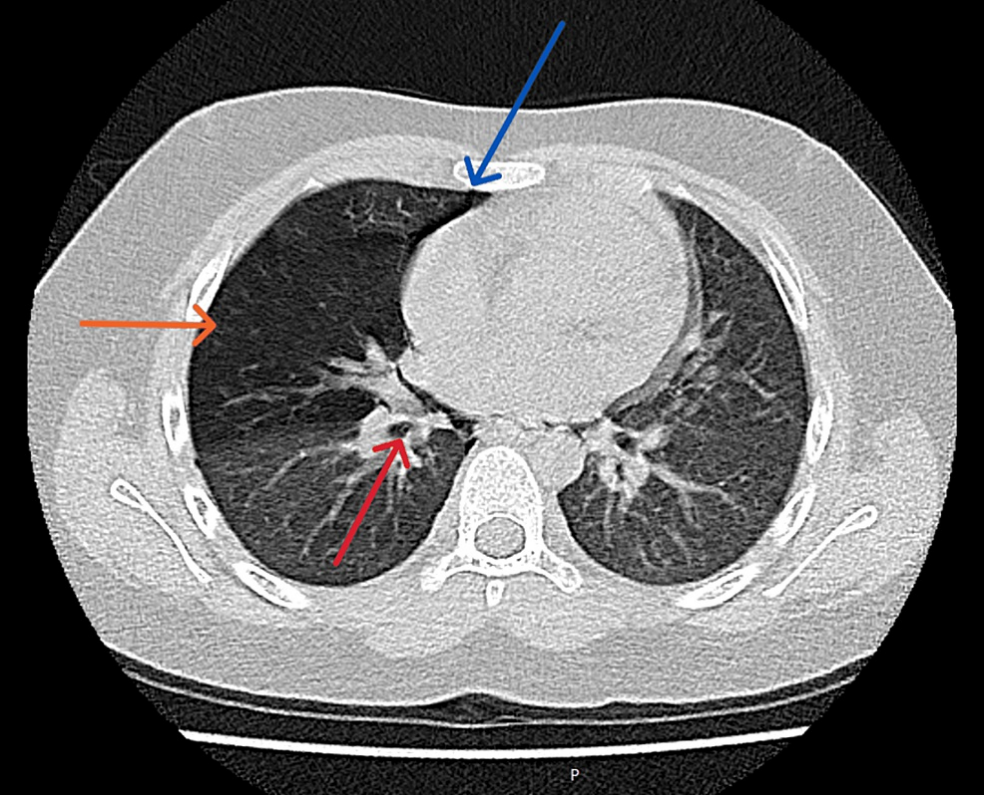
A slice of CT (axial view) showed the foreign body (red arrow), pneumothorax, pneumomediastinum (blue arrow), and middle lobe air trapping (orange arrow).
